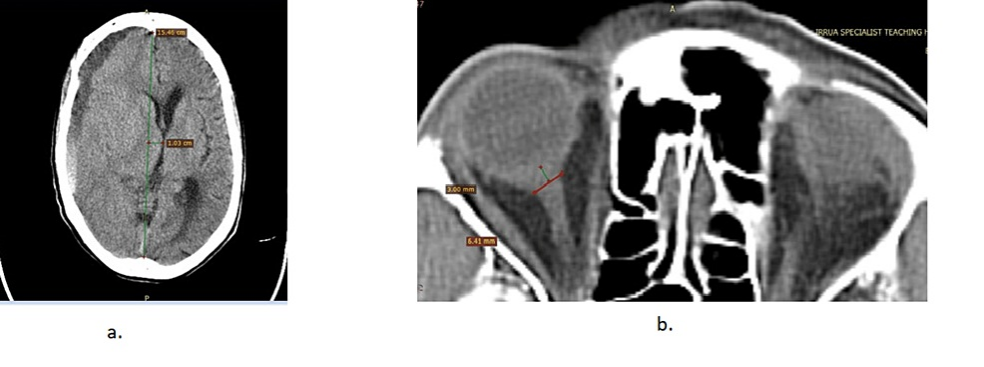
Non-enhanced CT images of the head. (a) There is an extensive crescentic lesion in the right parietal convexity, with a fluid-fluid (haematocrit) level. It has caused marked effacement of the regional sulci and the anterior horn of the right lateral ventricle, with significant contralateral shift (>5 mm) of the midline brain structures. (b) At the level of the orbit, there is increased ONSD (6.41 mm) taken 3 mm behind the globe. Only the right intraconal ONSD complex is fully shown in this section. ONSD: optic nerve sheath diameter.
